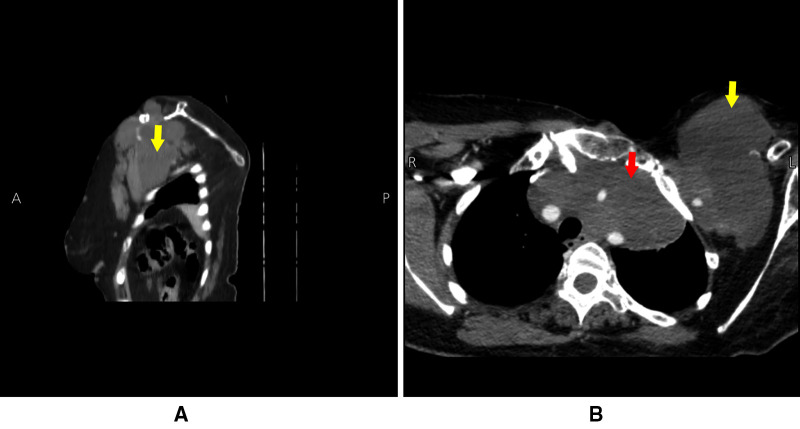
CT scans done in June 2021 showing a large lobulated hypodense mass lesion. Red arrow indicates the mediastinal lesion, yellow arrows indicate the extrathoracic chest wall lesion.
